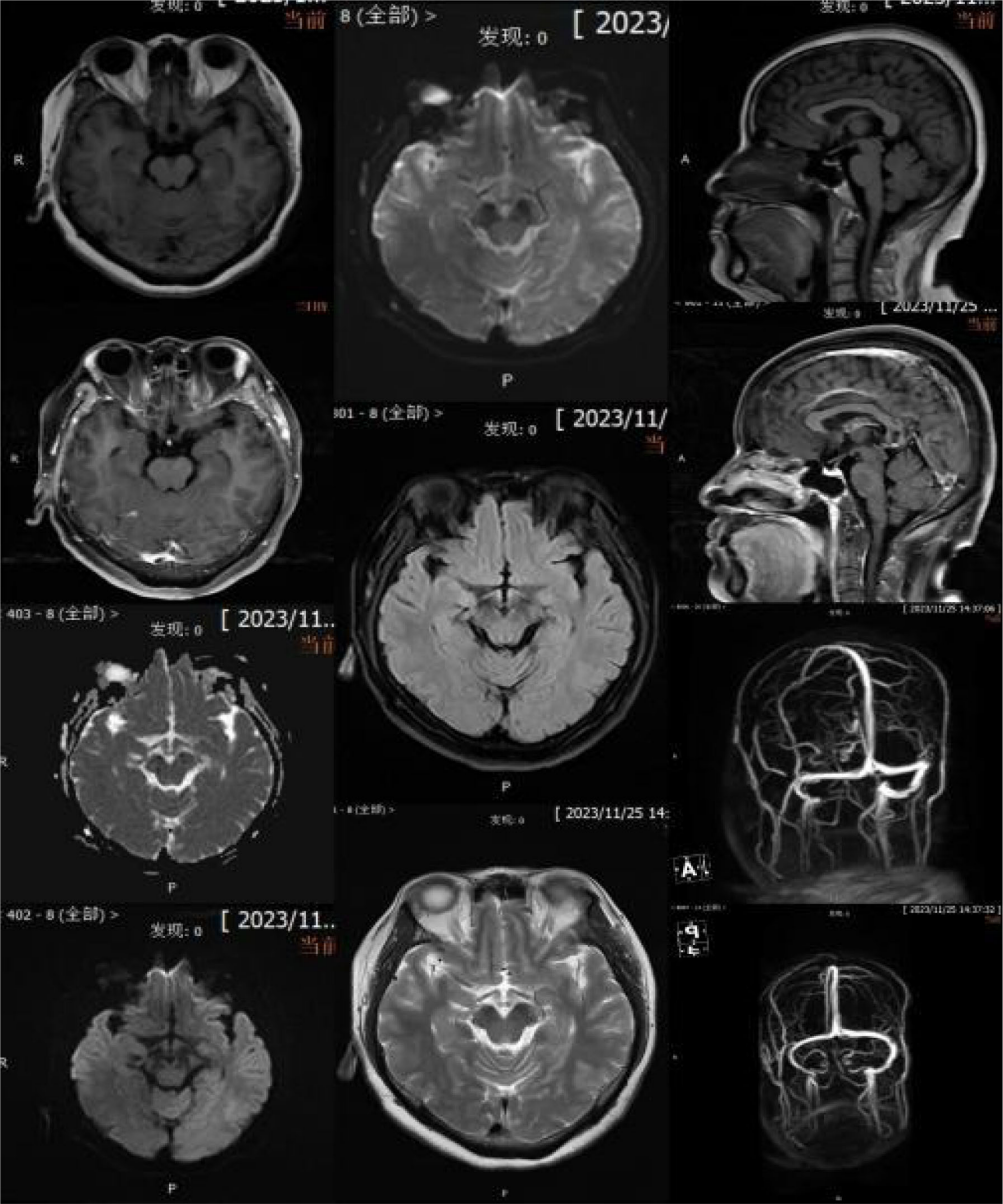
Brain MRI scan + contrast-enhanced + MRV.
